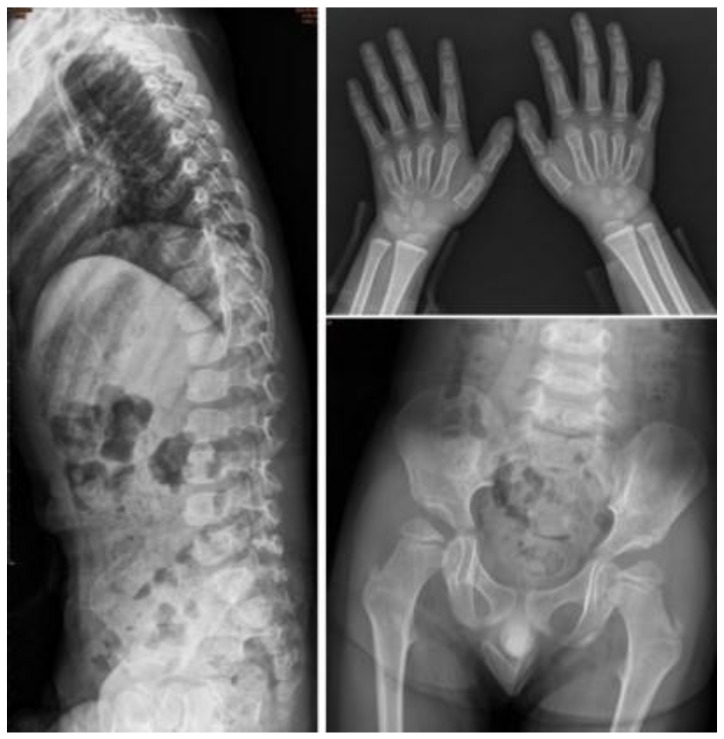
The bone X-rays at 3.2 years of age of patient No. I-5 who had the variation allele c.1400C > T [p.P467L]. He received ERT at 0.5 years of age. At 3.2 years of age, rounded and anterior beaking of lumbar spine vertebral bodies, mild proximal tapering of metacarpal bones with bullet-shaped phalanges, round iliac wings, and inferior tapering of the ilea with not-well-developed acetabulum were found.
